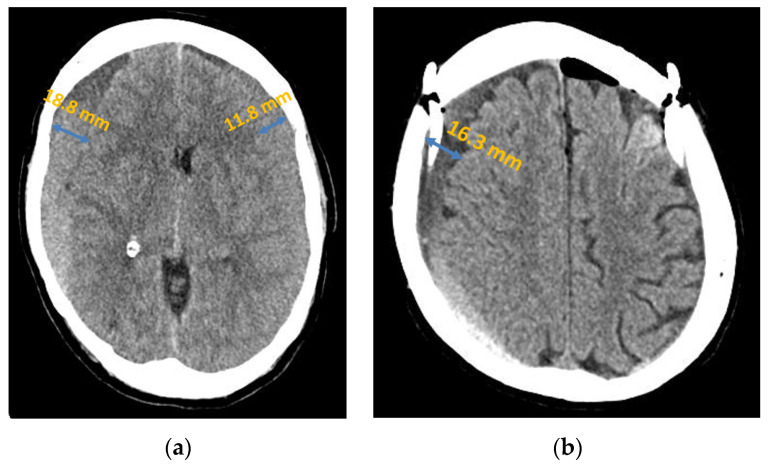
Chronic subdural haematoma extended over both hemispheres: (a) preoperative presentation in axial CT; (b) postoperative presentation in axial CT.
